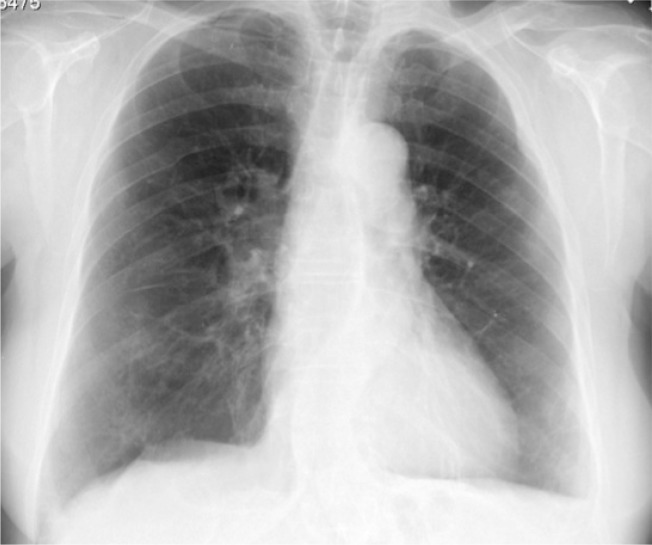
Chest X-ray at admission, showing a hypertransparent area in the upper mediastinum.
